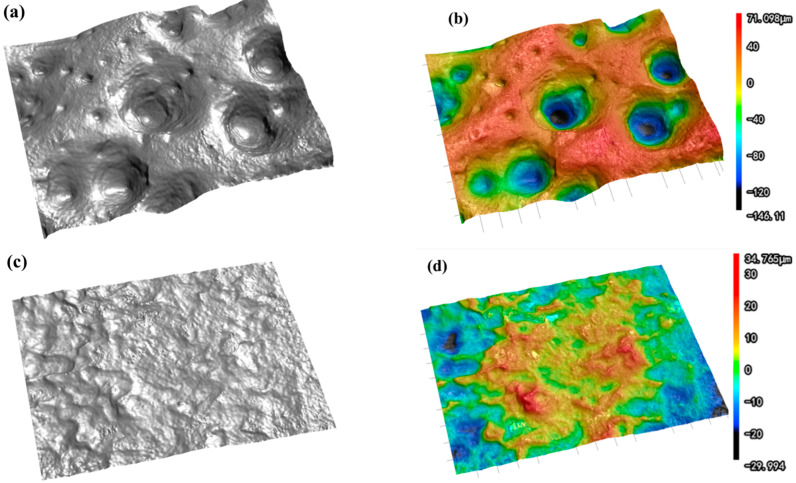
Optical profilometer scans of the tin sheets after ultrasonic treatment (a,b) and after conventional treatment (c,d).
